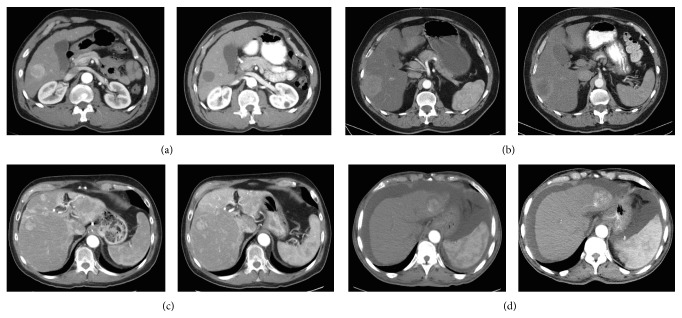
Axial CT images demonstrating the four mRECIST categories. (a) Complete: 100% HCC tumor necrosis. (b) Partial: 30%–99% HCC tumor necrosis. (c) Stable: between 29% HCC tumor necrosis and 20% HCC tumor growth. (d) Progressive: >20% HCC tumor growth.
